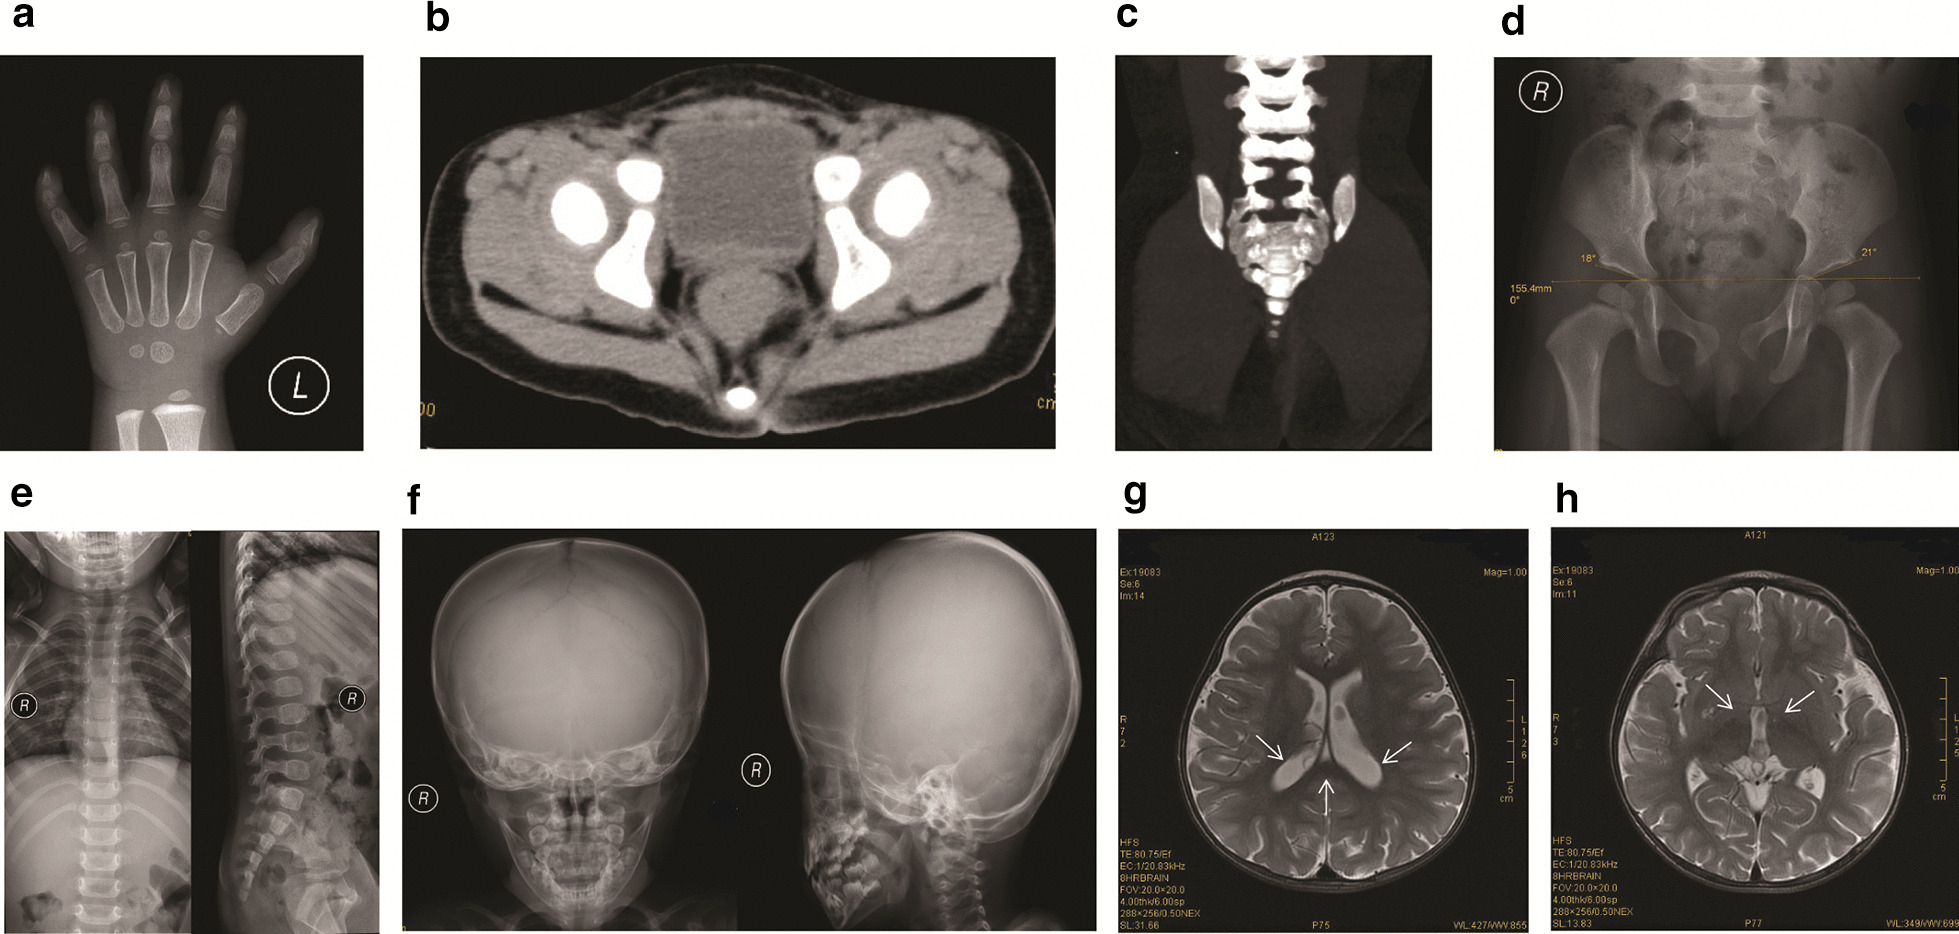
Imaging of the patient at the age of 2 years and 2 months. a Clinodactyly fifth finger. b Left-skewed caudal vertebra noted by the axial computed tomography (CT) scan. c Left-skewed caudal vertebra noted by the two-dimensional CT reconstruction. No abnormalities were noted in hip (d), main body of spine (e), and the skull (f). g Distended changes in bi-lateral ventricles (g) and the third ventricle (h), and the malformation of the sixth ventricles (g) were noted with the axial T2-weighted imaging. Arrows indicated the bi-lateral ventricles and the sixth ventricles in (g) and the third ventricle in (h)
